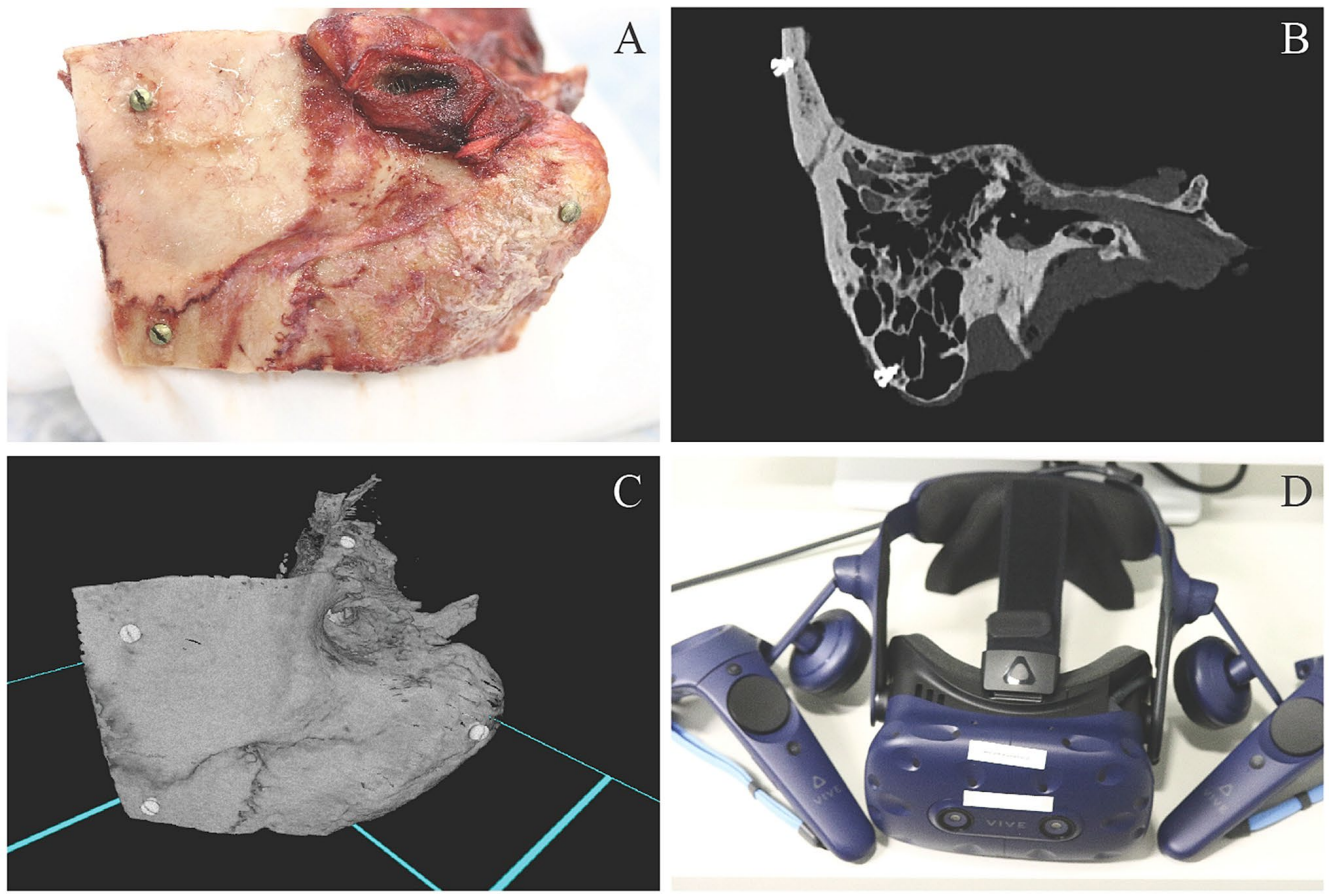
a Fresh frozen temporal bone, b Conventional 2D HRCT image of temporal bone, c Temporal bone in VR environment, d HTC Vive Pro head-mounted display and a pair of controllers
